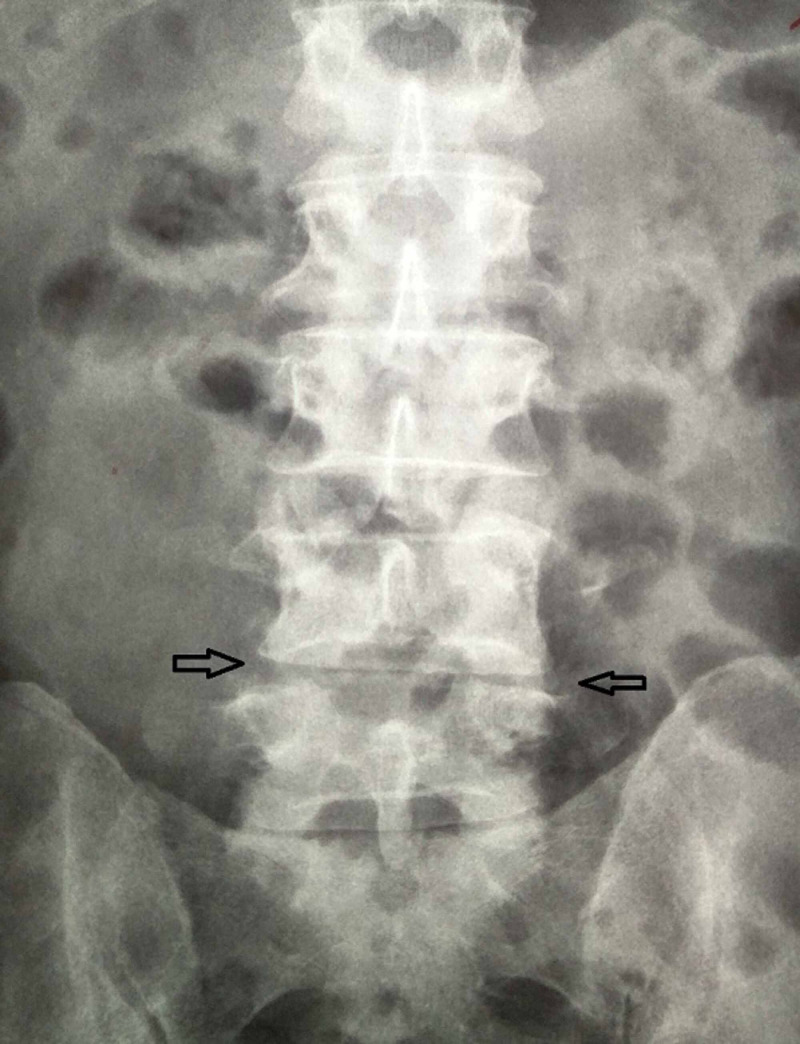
Preoperative anteroposterior roentgenogram of the lumbar spine showing narrowing of the intervertebral space (black arrows).
